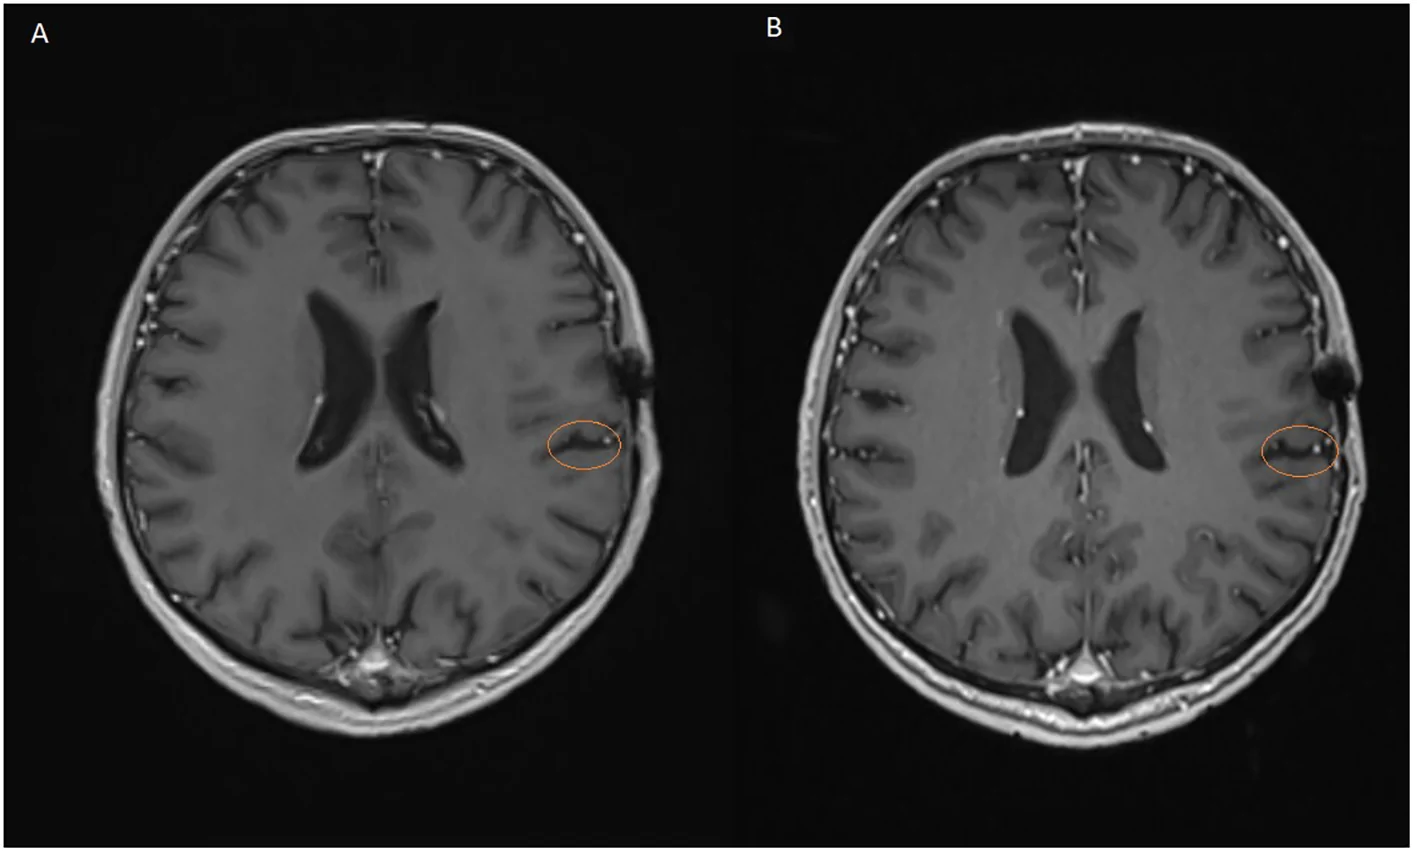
Follow-up contrast-enhanced T1-weighted MRI at 12 months (A) and 15 months (B) post-surgery showing stable post-operative changes without evidence of local recurrence (area marked in orange).
